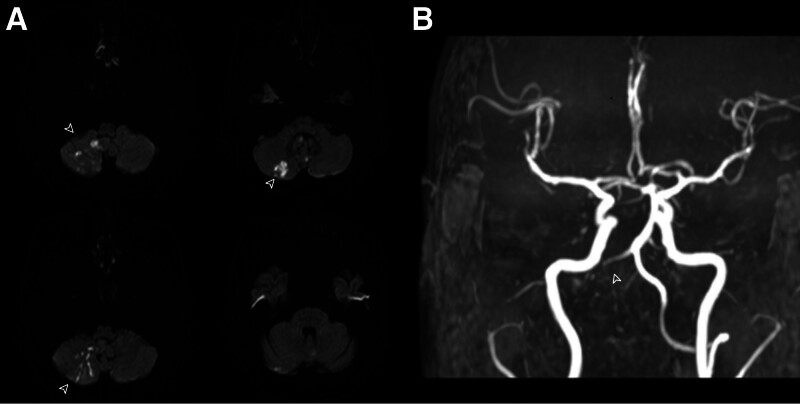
Neuroimaging of acute cerebellar infarction and vertebral artery occlusion. (A) Axial diffusion-weighted imaging (DWI; b = 1000 s/mm²): restricted diffusion in the right cerebellar hemisphere (arrow), predominantly involving the posterior inferior cerebellar artery (PICA) territory, consistent with acute infarction. (B) Magnetic resonance angiography (MRA; time-of-flight sequence): complete occlusion of the right vertebral artery (arrow).
